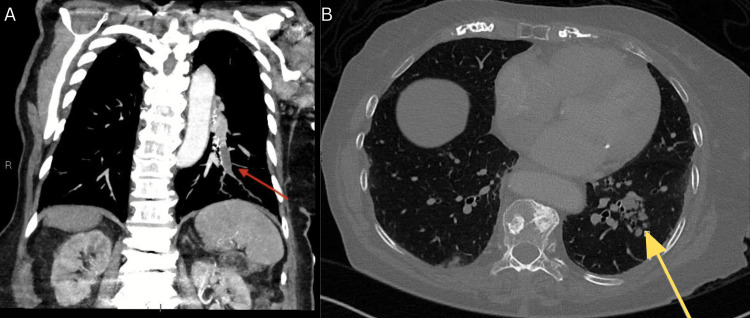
Initial CT of the chest post-contrast phase: (A) coronal maximum intensity projection view in soft tissue windows and (B) axial view. Coronal CT image demonstrates an occlusive filling defect in the left lateral basal segmental pulmonary artery with a distended lumen (red arrow). Axial CT image showing a cluster of nodules with a tree-in-bud pattern evident in the lateral basal segment of the left lower lobe (yellow arrow).
